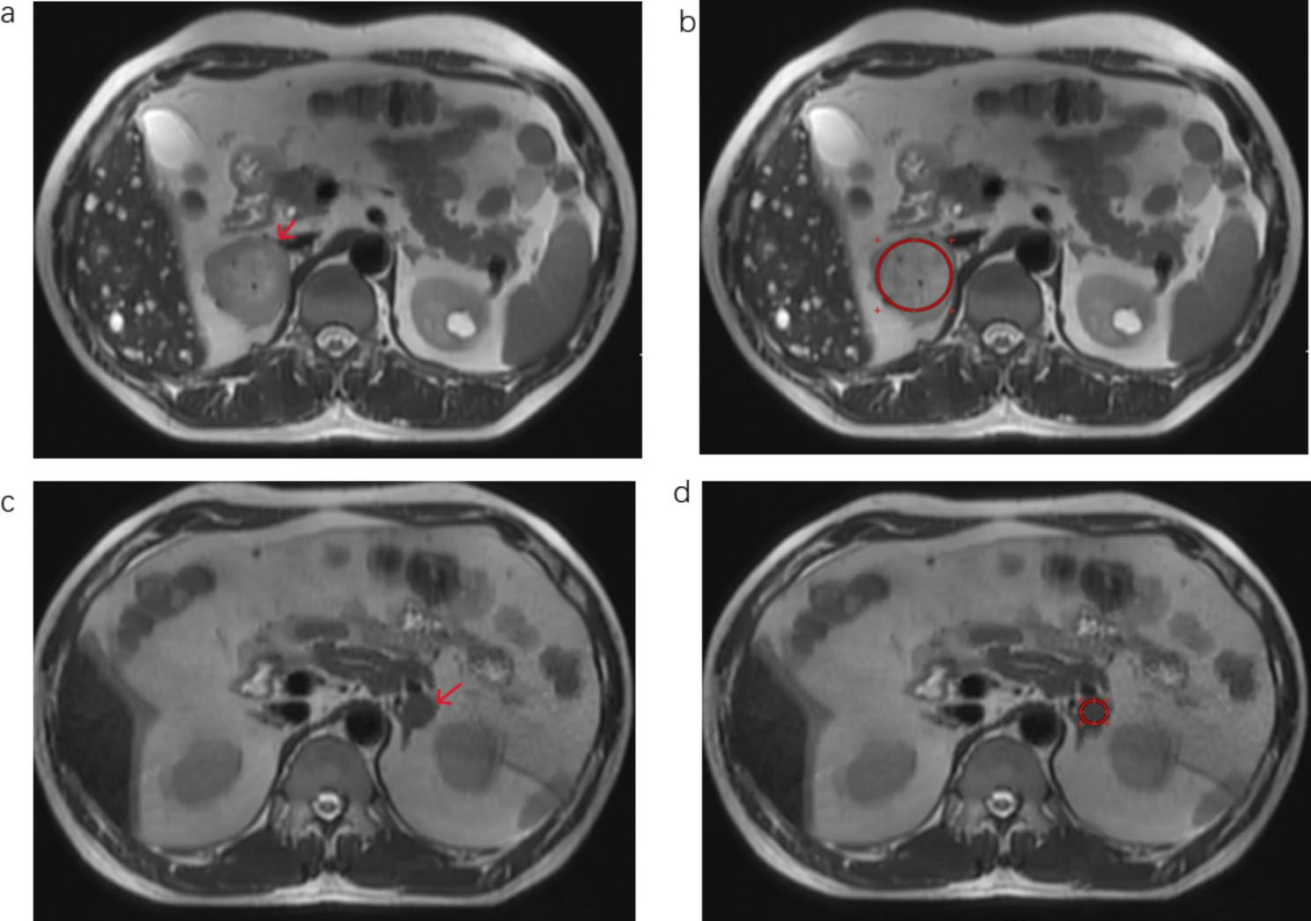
MR images in a man with right 5.2 cm pheochromocytoma(a-b) and in a man with left 1.7 cm adrenal adenoma (c-d). (a) Axial T2-weighted single-shot turbo spin-echo image depicts the right adrenal nodule (arrow) with high signal intensity (SI). (c) Axial T2-weighted single-shot turbo spin-echo image depicts the left adrenal nodule (arrow) with low signal intensity (SI). (b, d) shows method of measurement of T2-weighted SI ratio. Circular (red) region of interest (ROI) depicts method of measurement of adrenal T2-weighted SI for homogeneous nodules. A ROI was placed in the ipsilateral skeletal muscle (not shown) to measure the adrenal-to-muscle T2-weighted SI ratio
